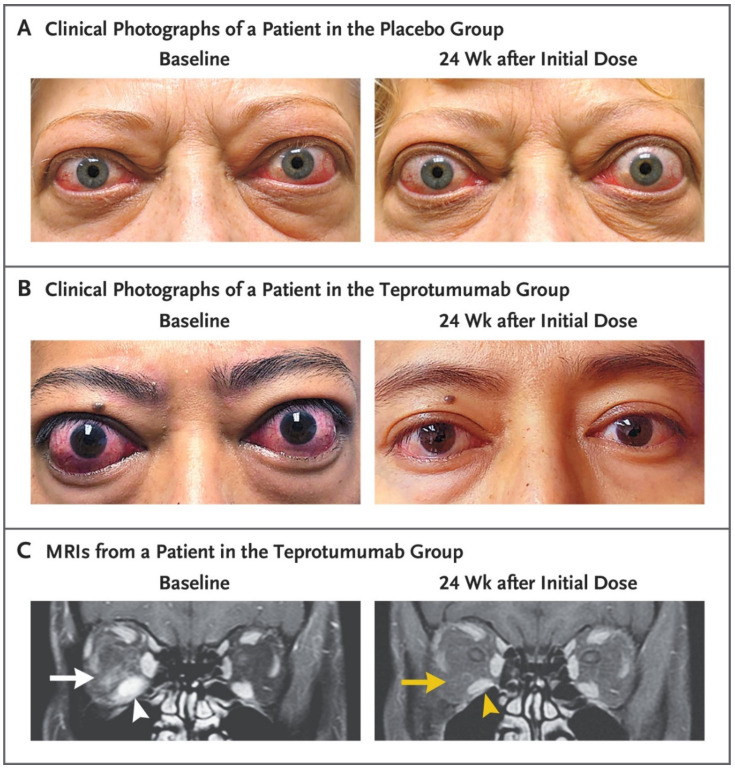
Photographs and MRIs at Baseline and 24 Weeks after receiving either placebo or teprotumumab in the Phase 3 trial. (A) Facial images of a patient receiving placebo. At baseline, substantial proptosis (left eye, 29 mm and right eye, 27 mm) and inflammatory signs (left eye Clinical Activity Score of 7 and right eye 5) are present. At week 24, proptosis and inflammation remain. (B) Facial images of a patient receiving teprotumumab. At baseline, proptosis (both eyes 24 mm), edema, upper and lower eyelid retraction, and multiple inflammatory signs (CAS 5 bilaterally) are present. At week 24, bilateral reductions in proptosis (−5 mm) and CAS (−4 points). (C). Contrast-enhanced, MRI shows fat-saturated T1-weighted coronal views in a single patient treated with teprotumumab at baseline and week 24. Enhancement of the inferior rectus muscle (white arrowhead) and orbital fat (white arrow) and nferior rectus muscle enlargement. At week 24, resolution of inferior rectus muscle (yellow arrowhead) enhancement and orbital fat (yellow arrow). Muscle volume was reduced by 49% (yellow arrowhead). Proptosis reduction decreased from 23 mm at baseline to 18 mm at week 24. From N. Engl. J. Med, Douglas R.S, Kahaly G.J., Patel A., Sile E.H.Z., Thompson R. et al. Teprotumumab for the treatment of active thyroid eye disease. 382; 341–352. Reprinted with permission from ref. [101]. Copyright 2020 Massachusetts Medical Society.
